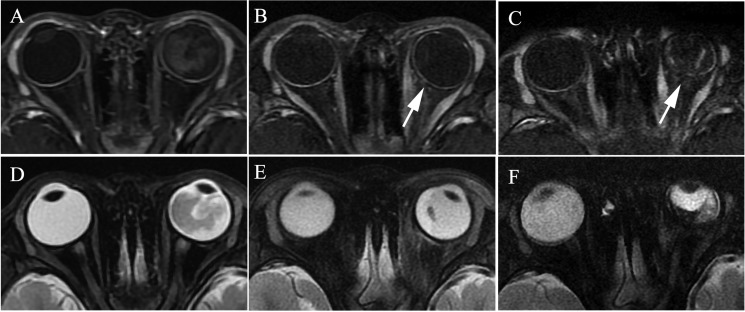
Retinoblastoma of the left eyeball in a 47-month-old male (patient 5) The axial contrast-enhanced T1-weighted, fat-saturated MRI (A–C) show regular enhancement of the choroid in the left eyeball (A), and decreasing and thinning of the choroid was present when compared with the contralateral eyeball in the first month after two cycles of IAC (B). Intermittent and irregular enhancement of the choroid was present in the fifth month after IAC (C). (D–F) The axial T2-weighted MRI show a progressive shrinkage of the left globe after IAC.
